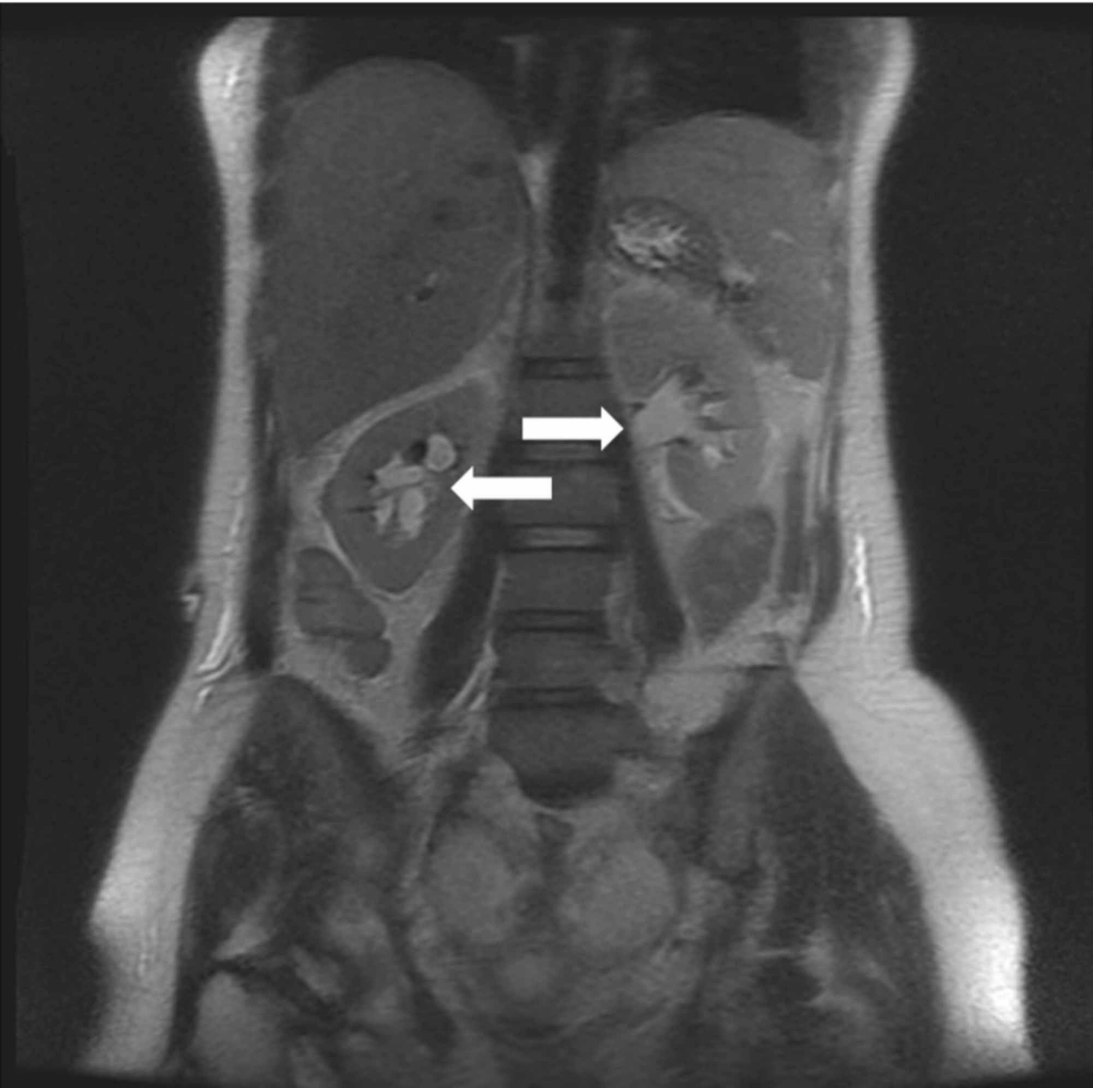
Coronal T2-weighted MRI of the abdomen demonstrating dilatation of the collecting systems (arrows), confirming hydronephrosis. MRI, magnetic resonance imaging
